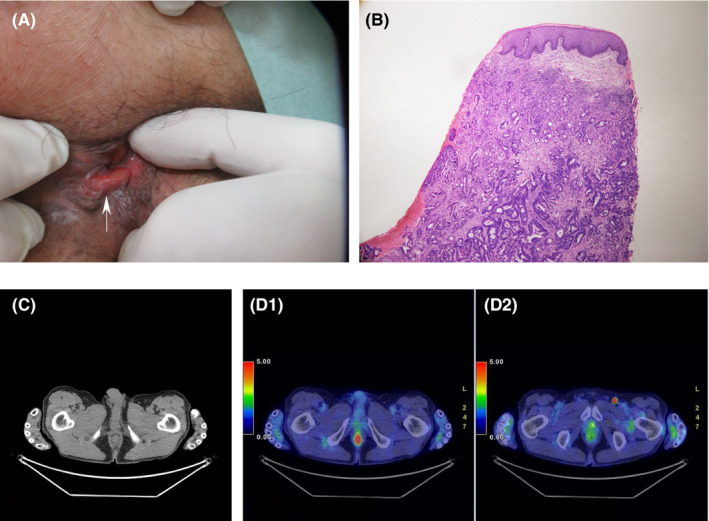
(A) An anal examination reveals a pink, firm anal tumor. (B) Biopsy sample shows the heterotypic columnar epithelium arranged in irregular duct‐like configurations, amalgamation duct‐like configurations, and comb‐like configurations under the stratified squamous epithelium. (C) Conventional CT did not reveal an anal tumor. (D) A positron emission tomography scan reveals an anal tumor (D1) and an inoperable left inguinal lymph node metastasis (D2)
